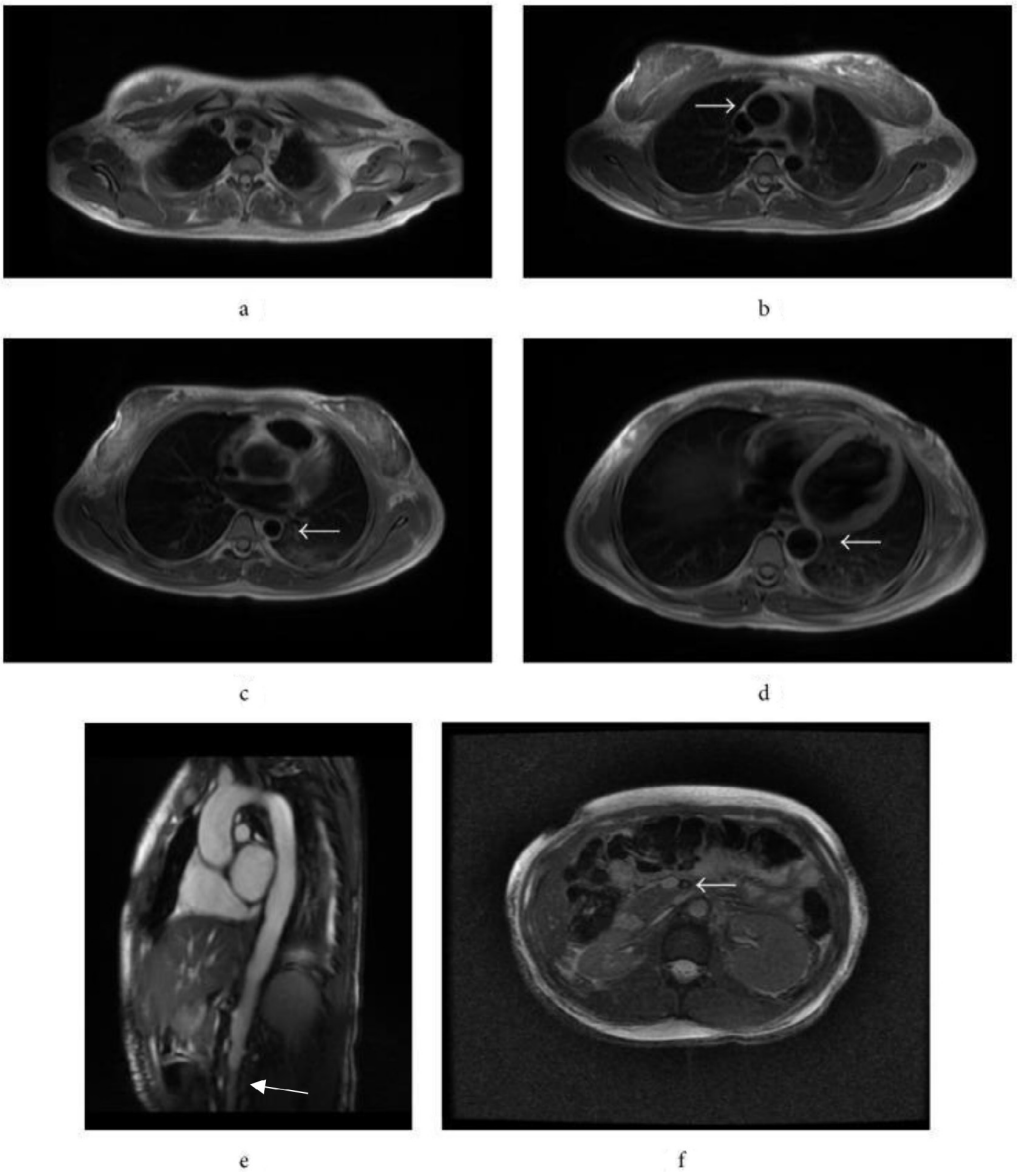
(a) Mural thickening of the brachiocephalic, left common carotid, and left subclavian arteries as seen on MRI/MRA of the chest, abdomen, and pelvis. (b). Similar study demonstrating mural thickening of the ascending aorta, measuring 3.4 cm in diameter. (c). Focal narrowing of descending aorta, measuring 2.2 cm. (d). Dilation of descending aorta distal to the proximal stenosis seen in 6c. (e). Irregular abdominal aorta vascular wall on sagittal cine-FIESTA sequence. (f). Thickening and mural narrowing of the superior mesenteric artery on axial T2 FIEST fat-saturated sequence. MRA, MR angiography.
